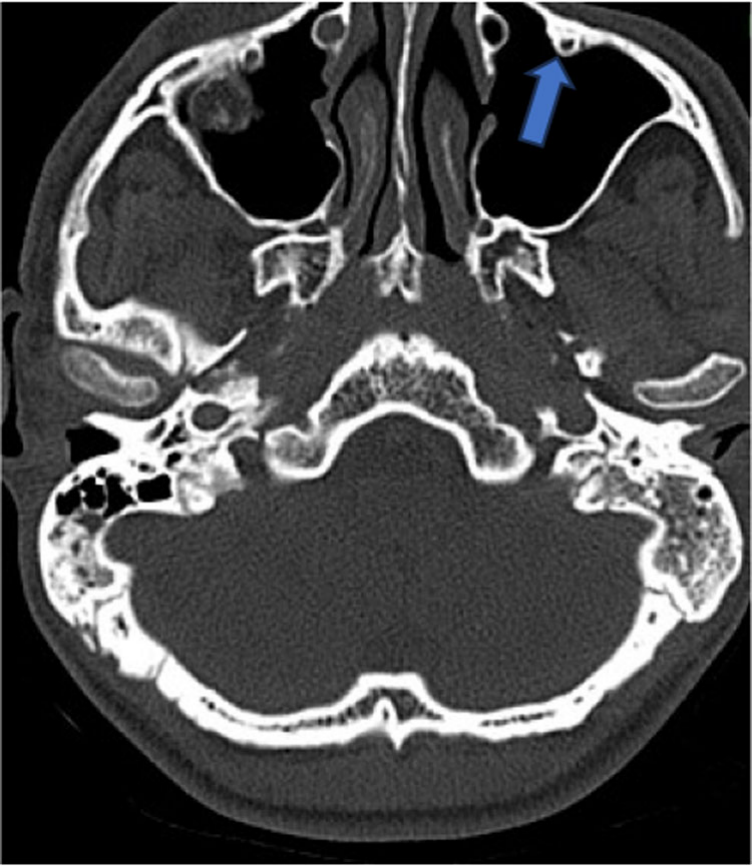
CT axial image shows exostosis of maxillary sinus.
